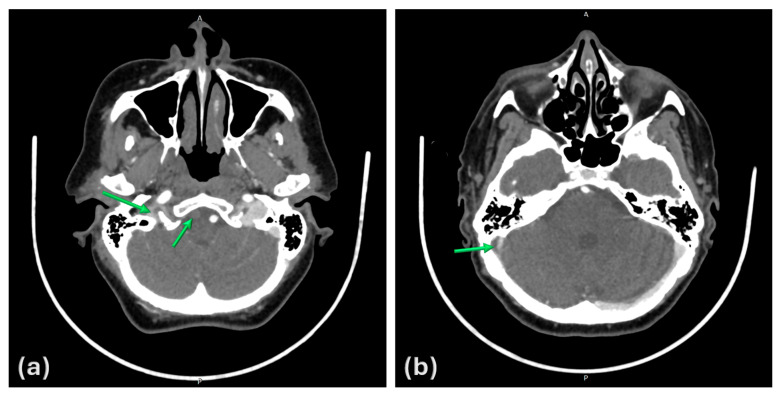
CT angiography shows thrombosis (arrows) in the right sigmoid sinus and occlusion in the V4 segment of the right vertebral artery (a) and thrombosis of the right transverse sinus (b).
